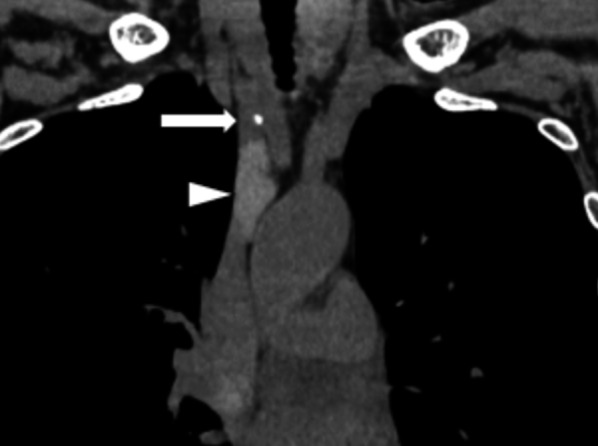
Chest computed tomography showing the right upper mediastinal mass containing a solid part of cephalic lesion (arrow) and a cystic part of caudal lesion (arrowhead). The tumor was located in the anterior side of the trachea and in the posterior side of the superior vena cava
